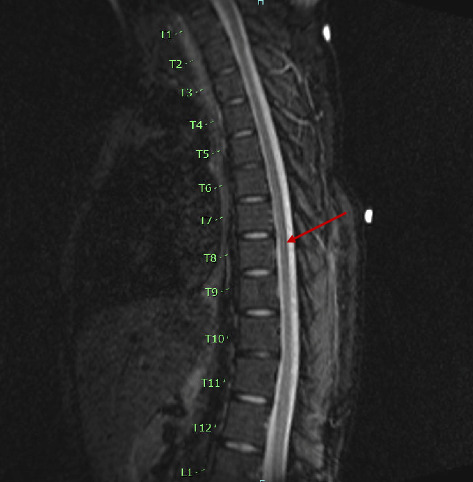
Magnetic resonance imaging of the thoracic spine demonstrating diffuse contrast enhancement of cauda equina nerve roots.
